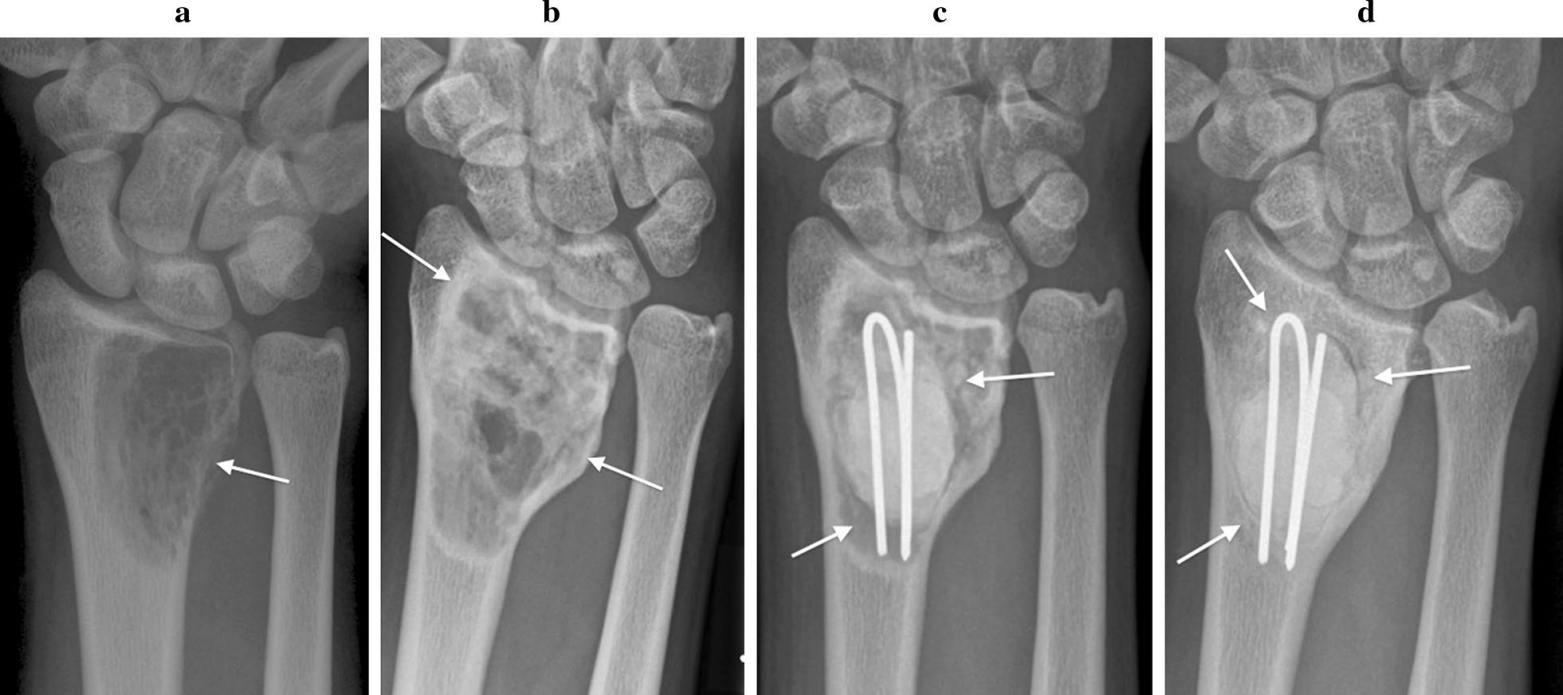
Patient 1: pre denosumab, a frontal plain radiograph shows a lytic Campanacci grade 2 distal radial GCTB with mild expansion and cortical thinning (arrow). Post denosumab at 2 months, b frontal plain radiograph demonstrates sclerosis of the tumour margin (arrows) and radial articular surface. There is mild modeling deformity of the radial articular surface and moderate internal matrix osteosclerosis. Post surgical, c frontal plain radiograph 1 week post surgical curettage, cementoplasty and internal fixation shows lucency of the peripheral tumour cavity (arrows) and d 2 years post surgery with progressive infilling of the lesion with osteosclerosis around the cement despite no post operative denosumab therapy (arrows)
